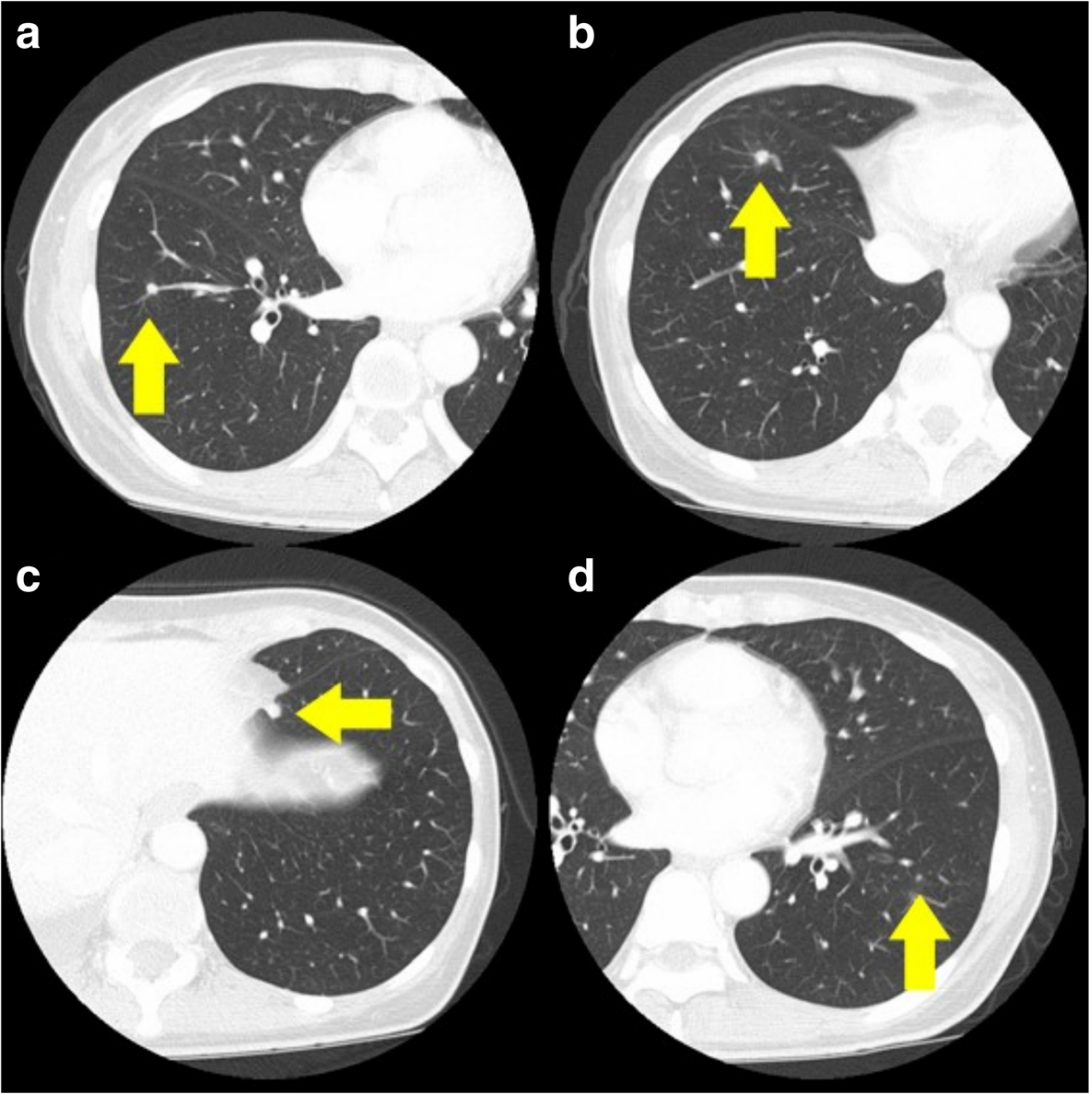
Preoperative CT images of abnormal nodules. a Right S8a (5 mm). b Right S8b (8 mm). c Left S9 (5 mm). d Left S8 (3 mm)
